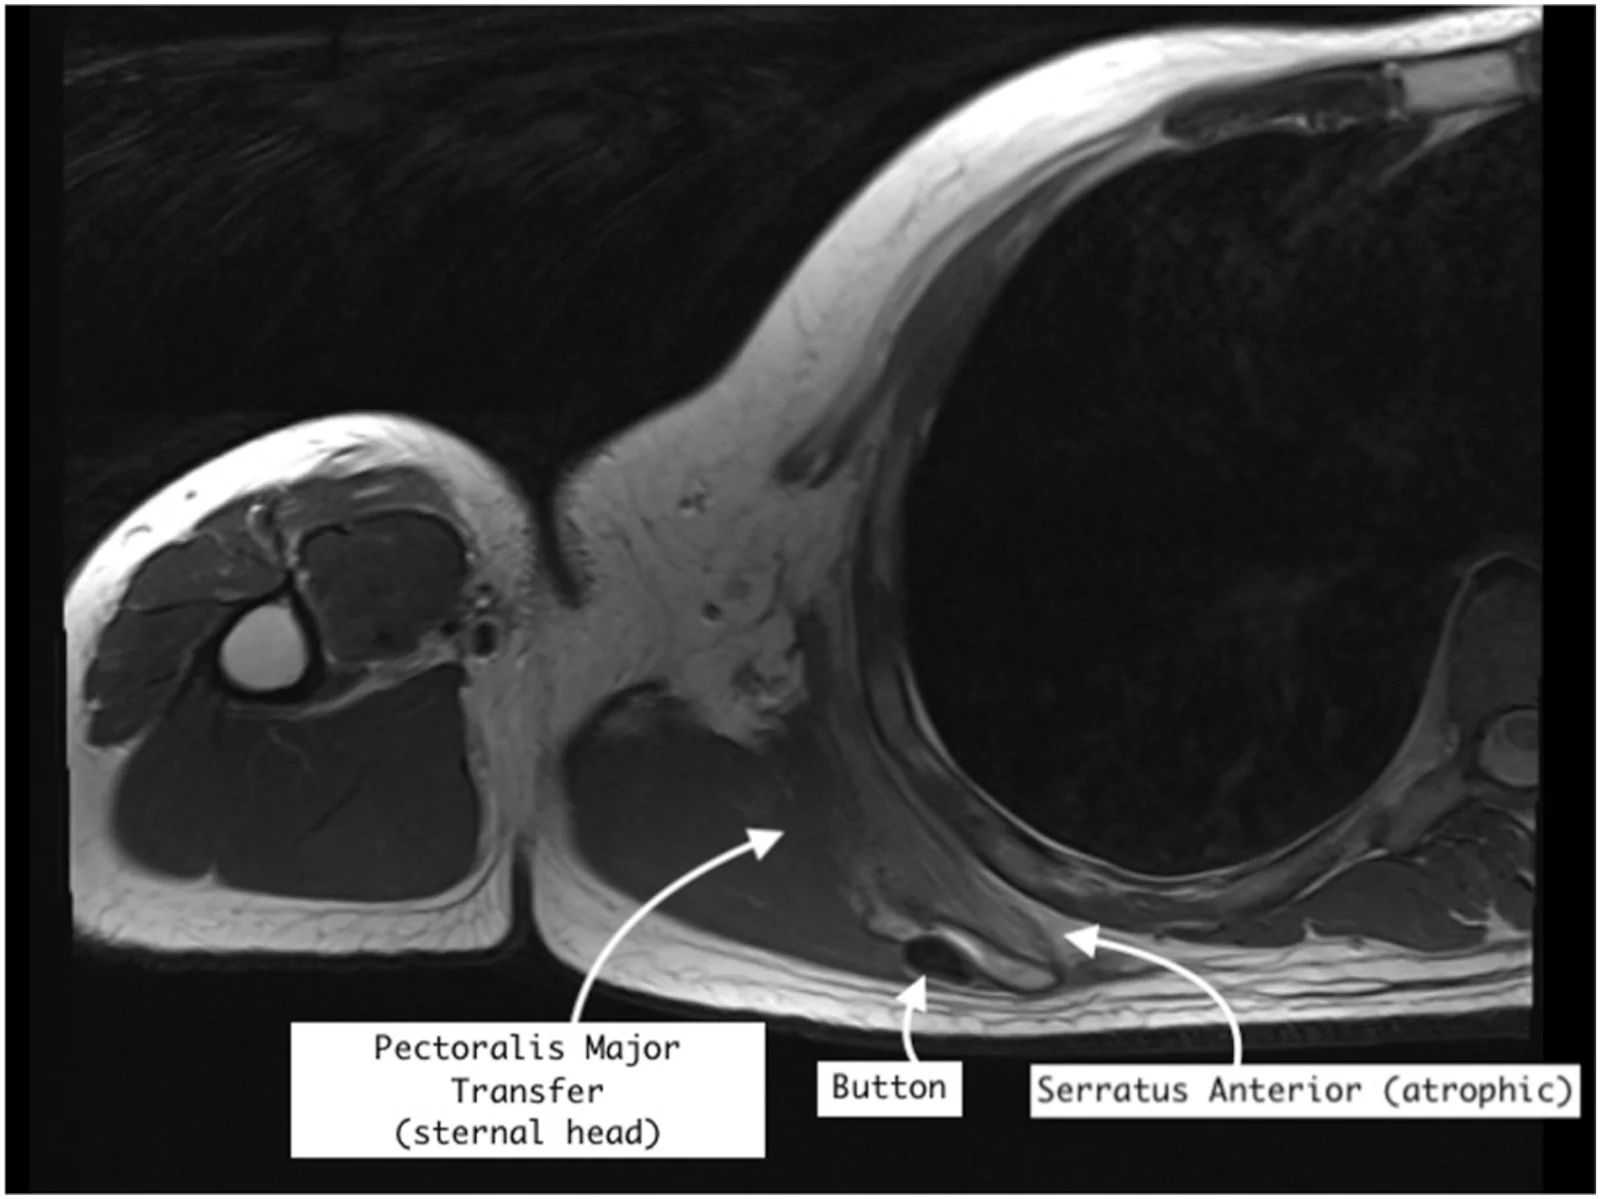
Axial T1-weighted magnetic resonance image of the thorax demonstrating the transferred pectoralis major tendon coursing toward its fixation at the inferior scapular angle.
